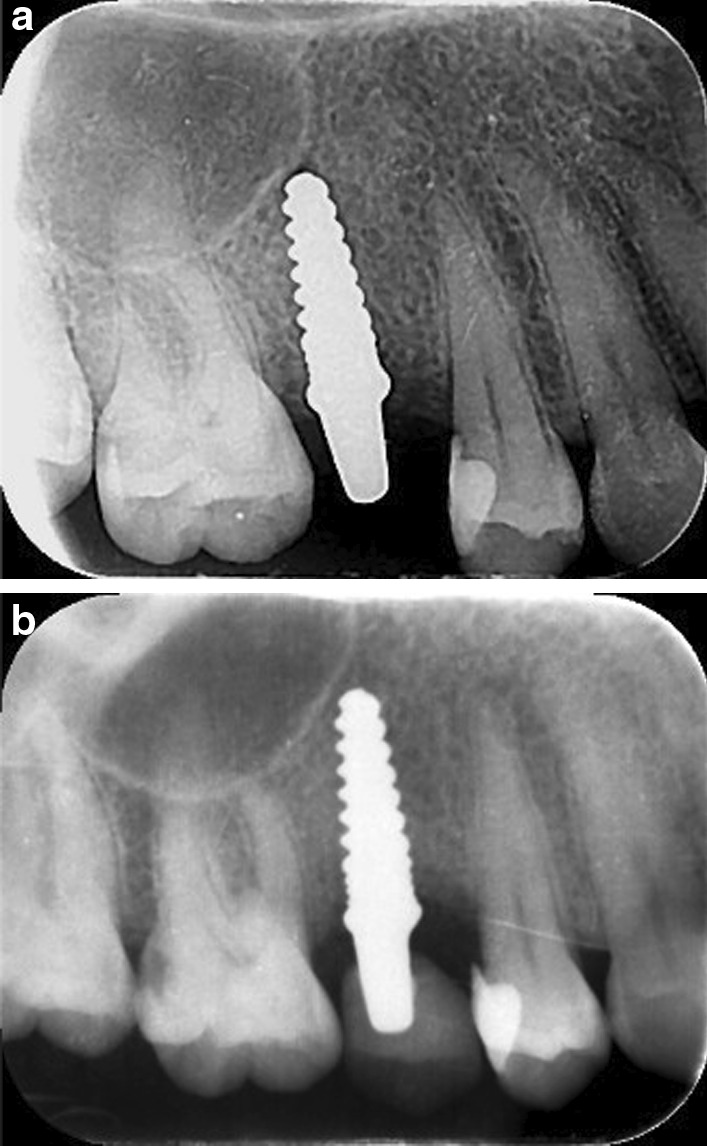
a Periapical radiographs of titanium implant after placement. b Periapical radiographs of titanium implant after 5 years
